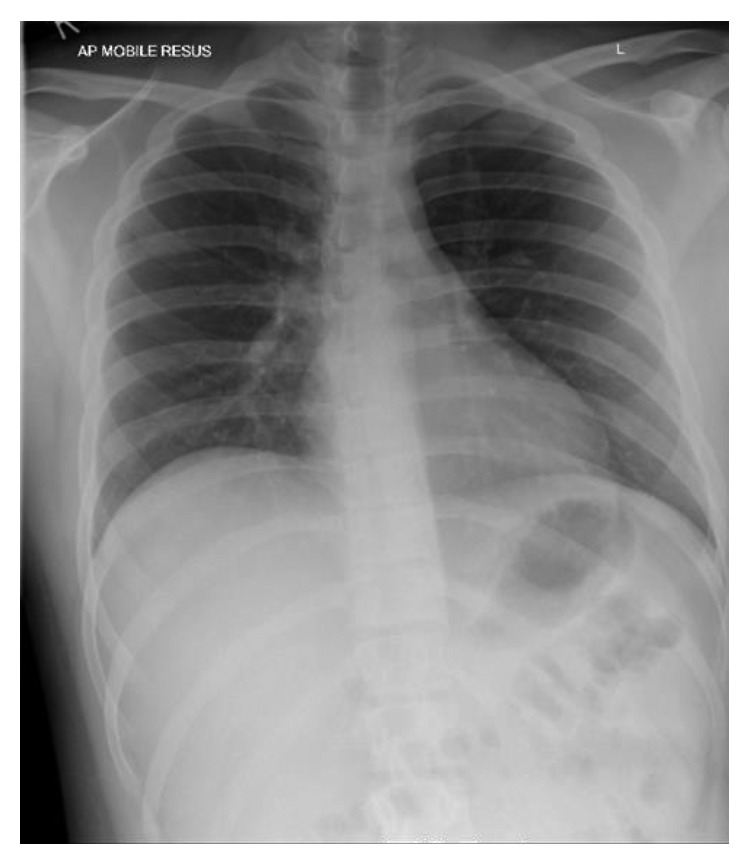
First chest X-ray (normal).
